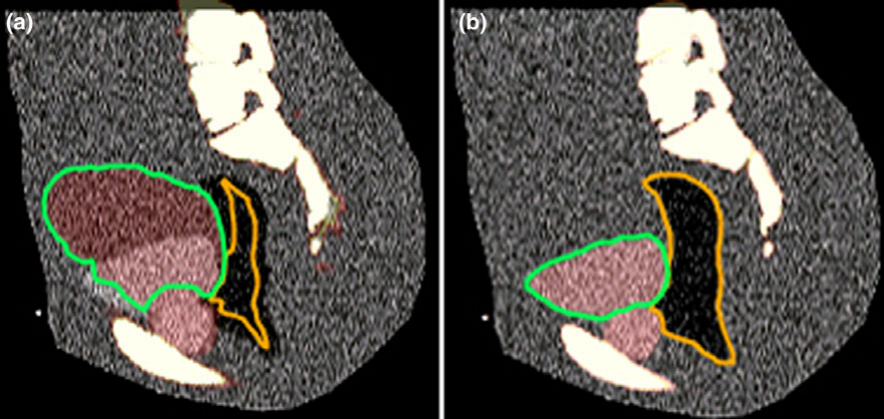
Deformable registration results for a noisy CT dataset (Case 15) with the Optical Flow (a) and Mutual Information (b) algorithms.
